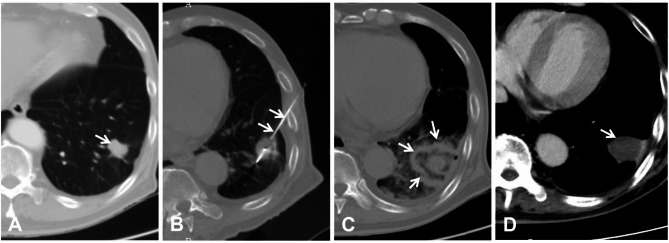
A 65-year-old male with left solitary PO from HCC, AFP: 98 ng/ml. (A) Pre-treatment CT showed left solitary PO with a diameter of 2.2 cm (arrow); (B) microwave applicator (arrow) was inserted into the PO; (C) The PO and its around 1.0 cm was completely ablated (40 W/3min) on CT (arrow); (D) Post-treatment CT at 4-month showed solid nodule involution without enhancement (arrow)
